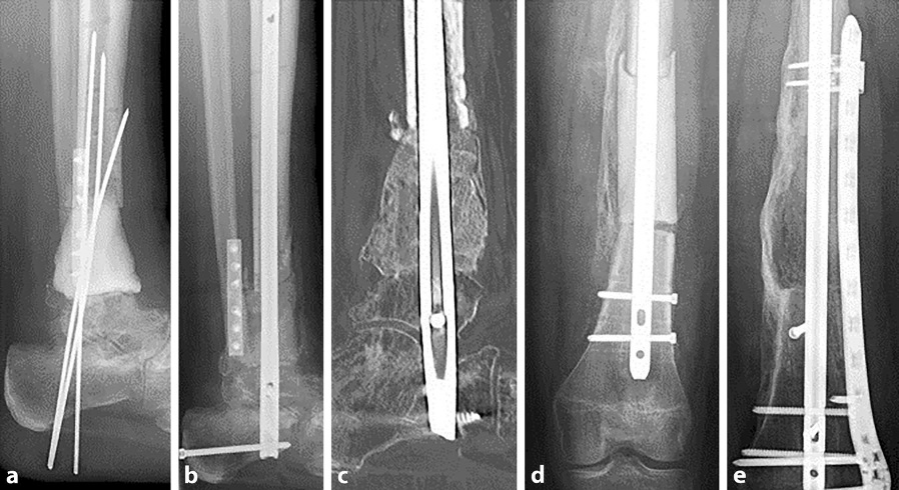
a Segmentdefekt der distalen Tibia mit Verlust des oberen Sprunggelenkes, Spacer und temporäre Stabilisierung mit Kirschner-Drähten, b MT mit 2 Femurköpfen und mittels RIA gewonnener Spongiosa, retrograde Marknagelarthrodese des Sprunggelenkes, c CT nach 4 Monaten mit vollständiger Konsolidierung; d 9 cm langer Segmentdefekt am Femurschaft, temporäre Osteosynthese durch Marknagel, e MT durch 2 Femurköpfe und RIA-Spongiosa, vollständige Konsolidierung nach 4,5 Monaten, hochstabile Osteosynthese durch Nagel und Plattenosteosynthese
